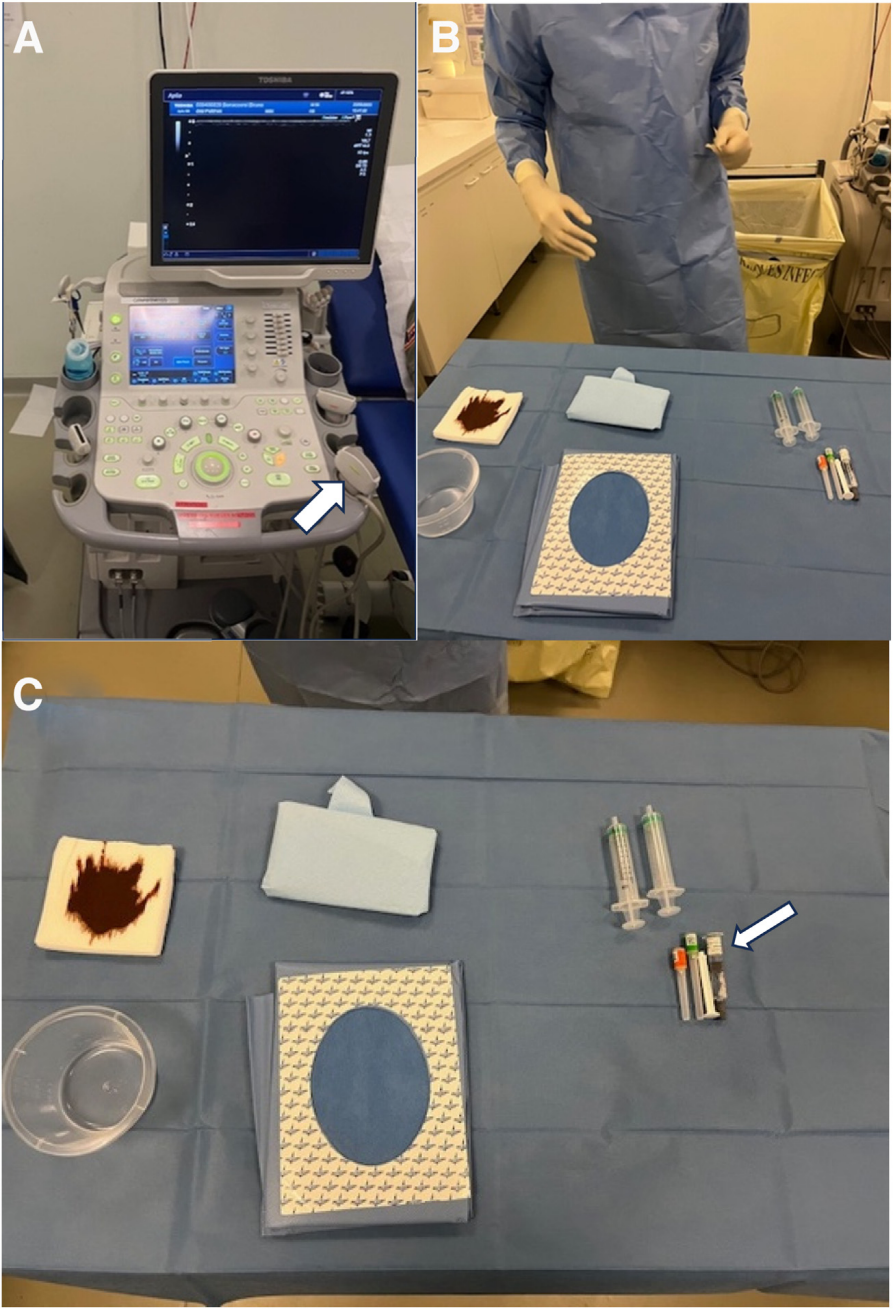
Equipment necessary for infiltration. (A) Toshiba ultrasound with a linear 18-MHz probe (white arrow). (B) Installation of the table under aseptic conditions. (C) View of the table: note that the material is very simple. We use 1 mL betamethasone and a 21-gauge needle for the infiltration (white arrow).
